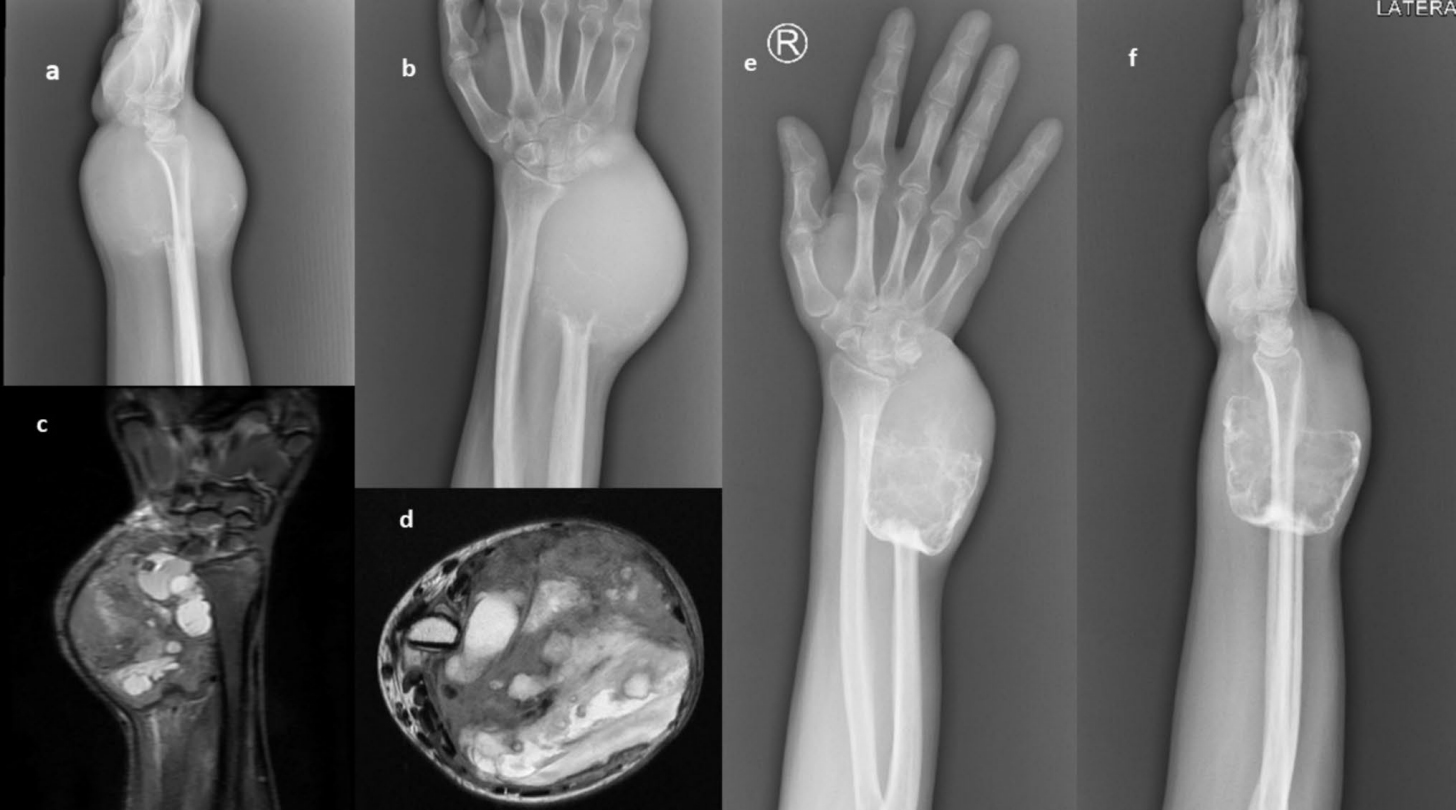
On initial presentation, a, b X-ray images showing eccentric lytic lesions and c, d MRI images showing soft tissue extension with cystic component. e, f X-ray images after denosumab adjuvant therapy with intralesional and peripheral sclerosis
